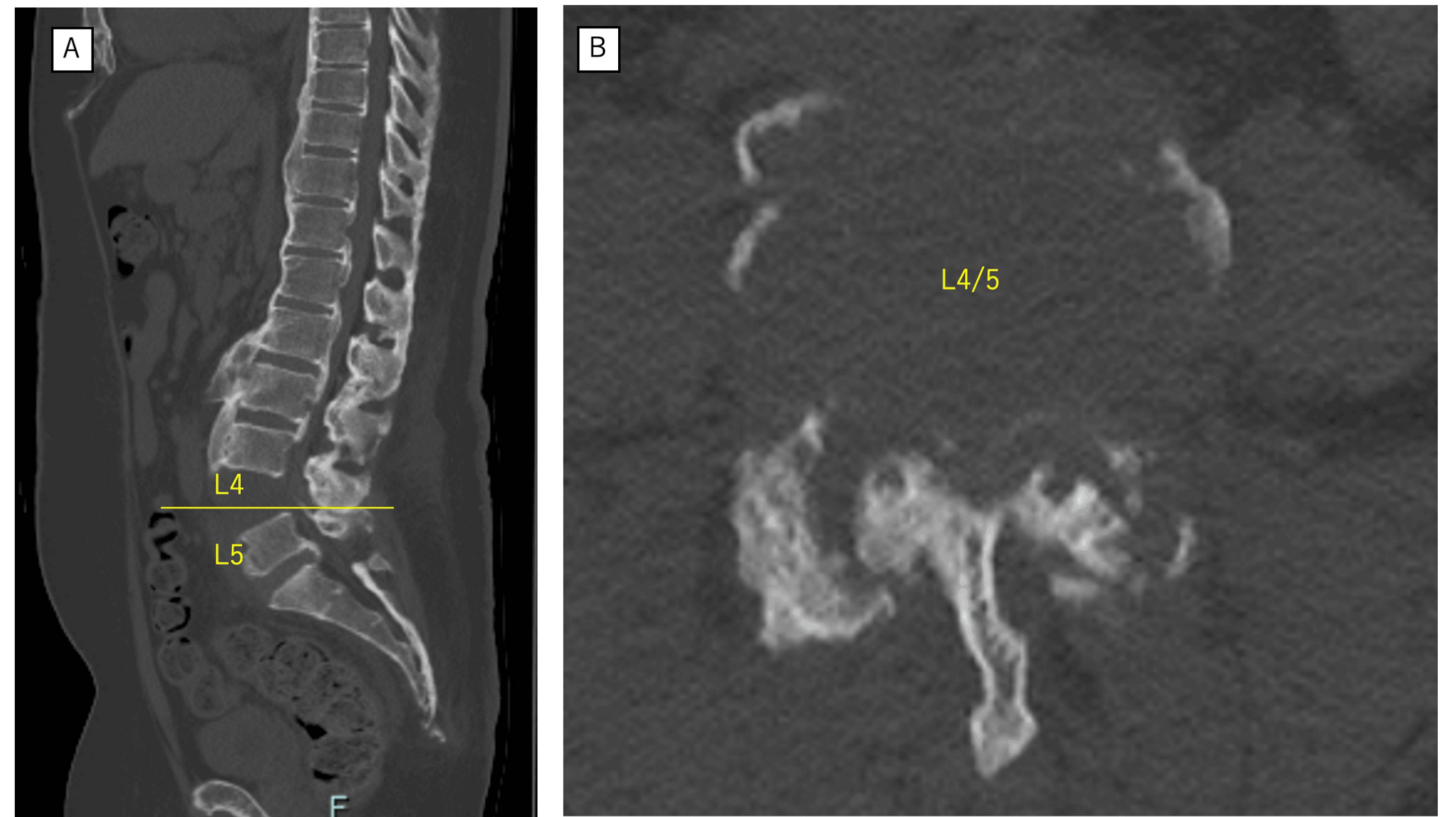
Preoperative computed tomography showing structural failure at L4/L5. (A) Sagittal reconstructed computed tomography image demonstrating extensive thoracolumbar ankylosis with spontaneous L4/L5 dislocation at the last mobile segment. (B) Axial computed tomography image at L4/L5 showing disruption of the posterior elements with facet separation.
